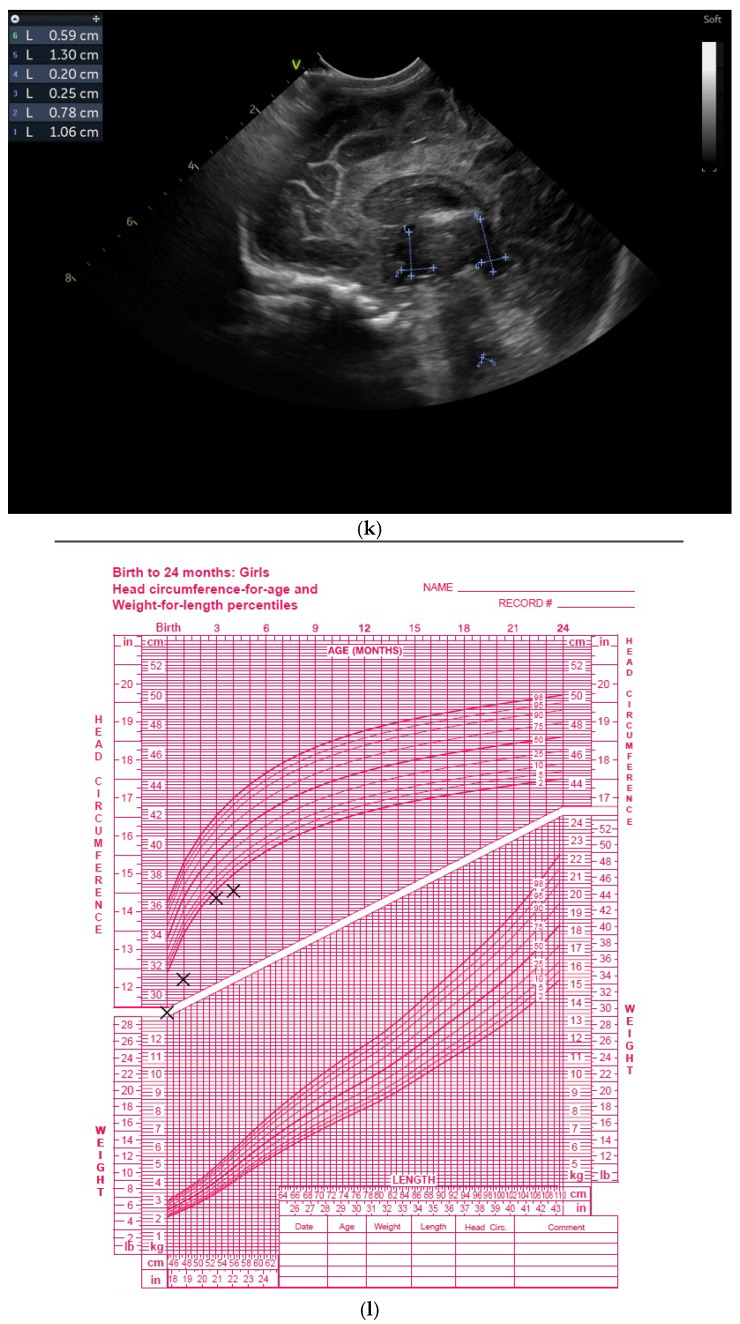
(a) Cranial ultrasound. Coronal view. Enlargement of the lateral ventricles with hyperechoic and third ventricle dilation. D1, D2—Levine index measurement. D3, D4—anterior horn width measurement. D5—third ventricle (personal image collection). (b) Cranial ultrasound. Sagittal view. Up—left ventricle. D1, D2—lateral ventricle measurements and thalamo-occipital horn width. Down—right ventricle. D1, D2—lateral ventricle measurements and thalamo-occipital horn width (personal image collection). (c) Cranial ultrasound. Sagittal view. Up—enlargement of the third and fourth ventricles. Measurements of the ventricles. Down—measurement of the resistive index (RI) performed on the anterior cerebral artery (ACA) using Doppler flow measurements. RI—0.74 (personal image collection). (d) Cranial ultrasound. Transtemporal view. Yellow arrow—enlargement of the Sylvian aqueduct (personal image collection). (e) Cranial ultrasound. Sagittal view. Yellow arrow—arachnoid cyst posterior to the third ventricle (personal image collection). (f) Cranial ultrasound. Coronal view. Lateral ventricles and third ventricle enlargement. D1, D2—Levine index measurement. D3, D6—right lateral ventricle measurement (anterior horn width). D4, D5—left lateral ventricle measurement (anterior horn width) (personal image collection). (g) Cranial ultrasound. Sagittal view. Left—left ventricle. D1—thalamo-occipital distance measurement. Right—right ventricle. D2—thalamo-occipital distance measurement (personal image collection). (h) Cranial ultrasound. Coronal view. Up—orange arrow—third ventricle. Red arrow—fourth ventricles. Yellow arrow—arachnoid cyst. Down—resistive index measured on the anterior cerebral artery. RI—0.79 (personal image collection). (i) Cranial ultrasound. Transversal view. Up—RI precompression value on the middle cerebral artery performed with Doppler examination. RI—68. Down—RI post-compression value on the middle cerebral artery performed with Doppler examination. RI—0.79 (personal image collection). (j) Cranial ultrasound. Up—coronal view. L1, L2—Levine index measurement of the lateral ventricles. Down—sagittal view. L1, L2—measurement of the thalamo-occipital distance (personal image collection). (k) Cranial ultrasound. Sagittal view. L1, L2—measurement of the third ventricle. L3, L4—measurement of the fourth ventricle. L5, L6—measurement of the arachnoid cyst (personal image collection). (l). Head circumference-for-age. “X” symbols represent the measurements performed from birth and follow-up examinations. Head circumference was still under the curve during follow-up examinations (personal image collection) [28].
